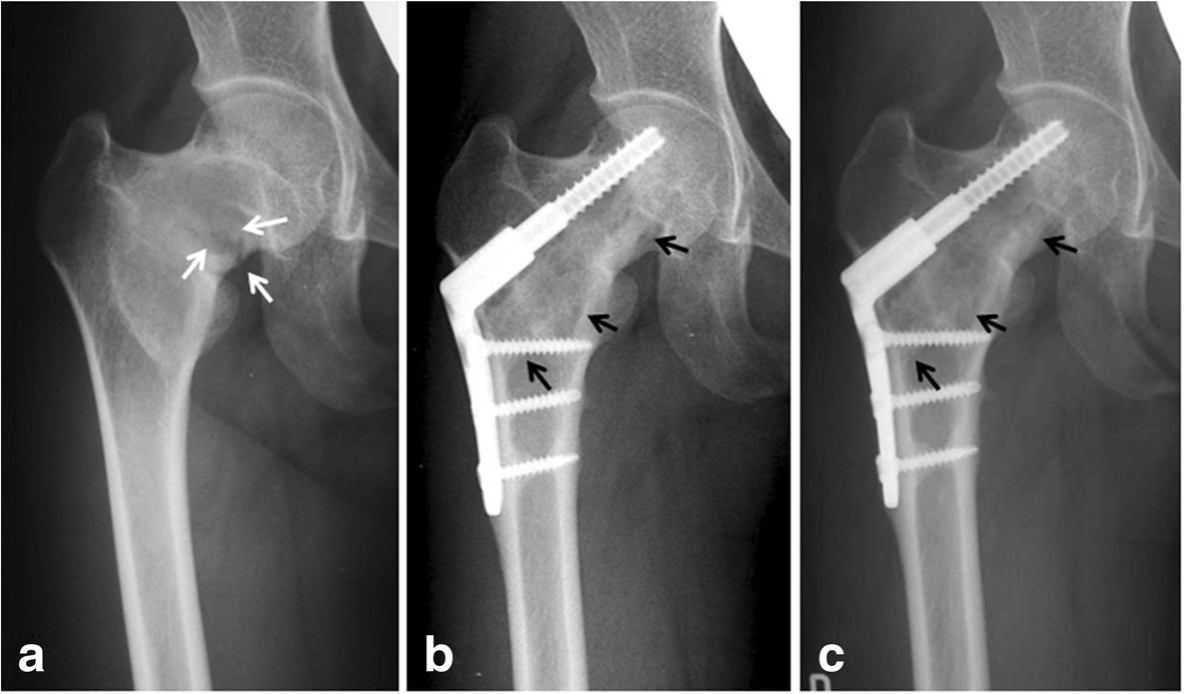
Patient 1. A 22-year-old female presented with repeated or persistent right hip pain since for ten years. An A-P X-ray at the initial presentation indicated fatigue fracture with “parrot’s beak” sign (*white arrows*) (a). X-rays at three years (b) and ten years (c) after surgery revealed cure of the fatigue fracture and preservation of the grafted autogenous fibular strut (*black arrows*)
